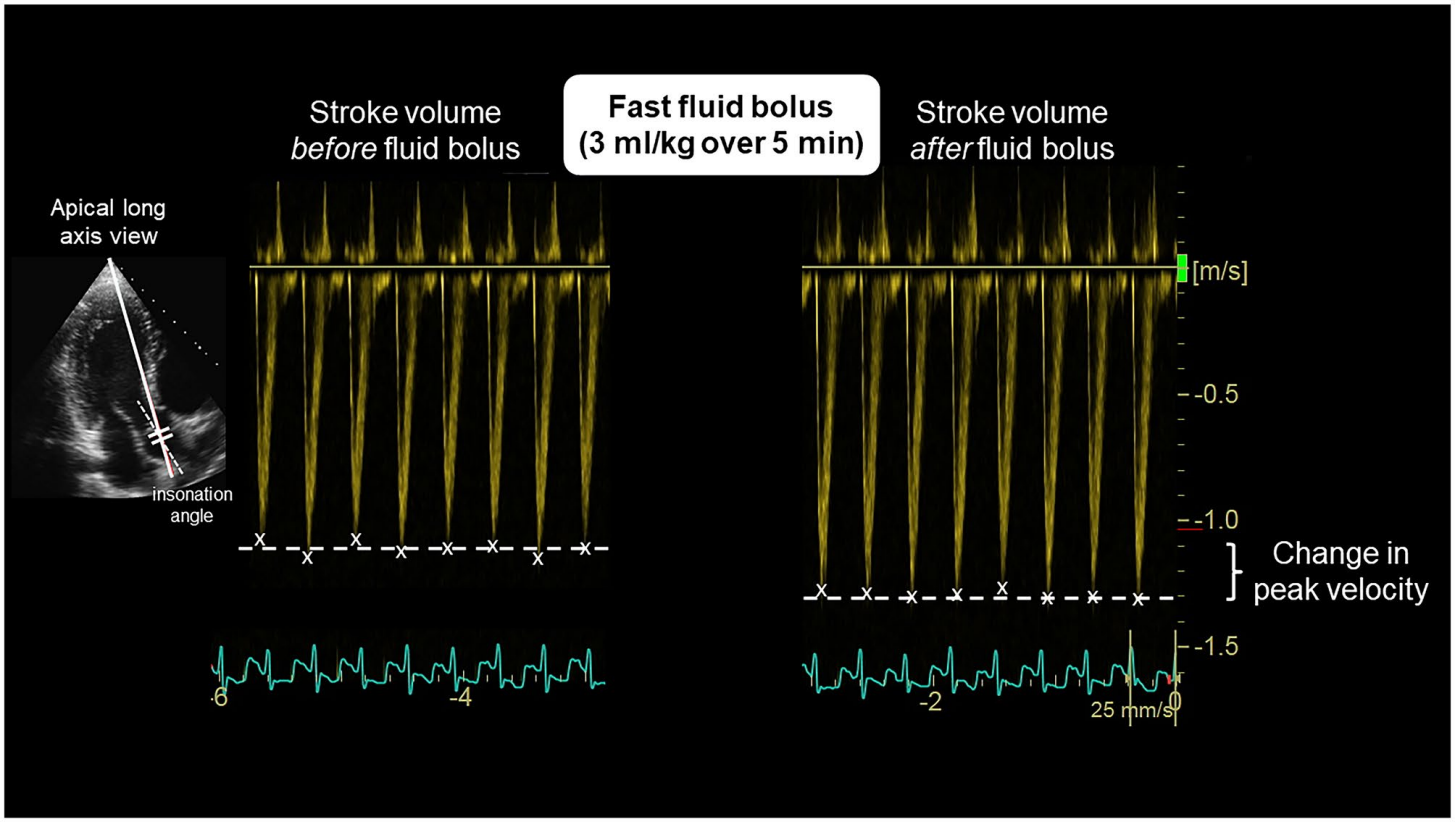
Example of a mini-fluid challenge (MFC) in a preterm infant. The apical long axis was used to visualise the aorta outflow tract. Pulse wave Doppler with minimal angle of insonation was added to capture stroke volume and determine the maximum velocity from 10 to 15 cardiac cycles at low sweep speed
